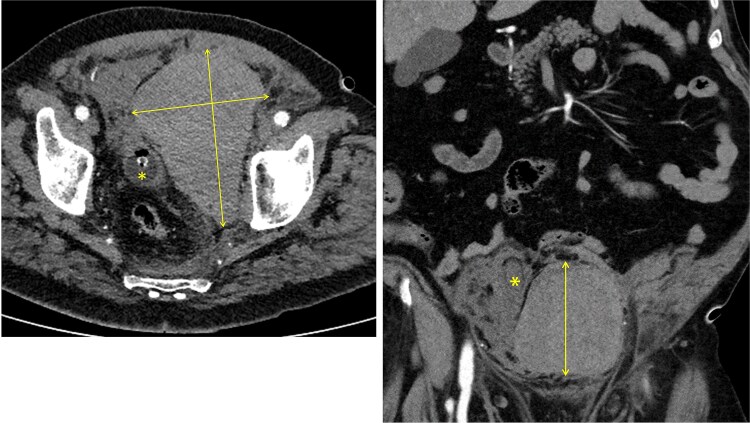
Left: axial CT showing a 14 × 9.5 cm extraperitoneal hematoma with urinary catheter balloon marked by an asterisk. Right: coronal CT showing an 11 × 9.5 cm extraperitoneal hematoma with the urinary bladder displaced to the right, indicated by an asterisk.
